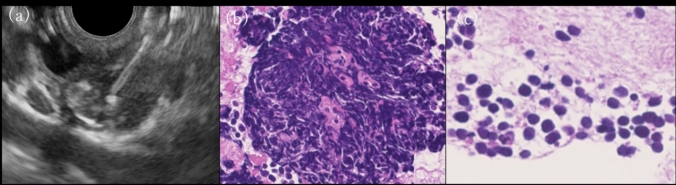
Endoscopic ultrasound-guided fine needle aspiration is performed on the tumor and nearby lymph nodes (a). Small tumor cells with high nucleocytoplasmic ratios are seen in both lesions. (b, c)
